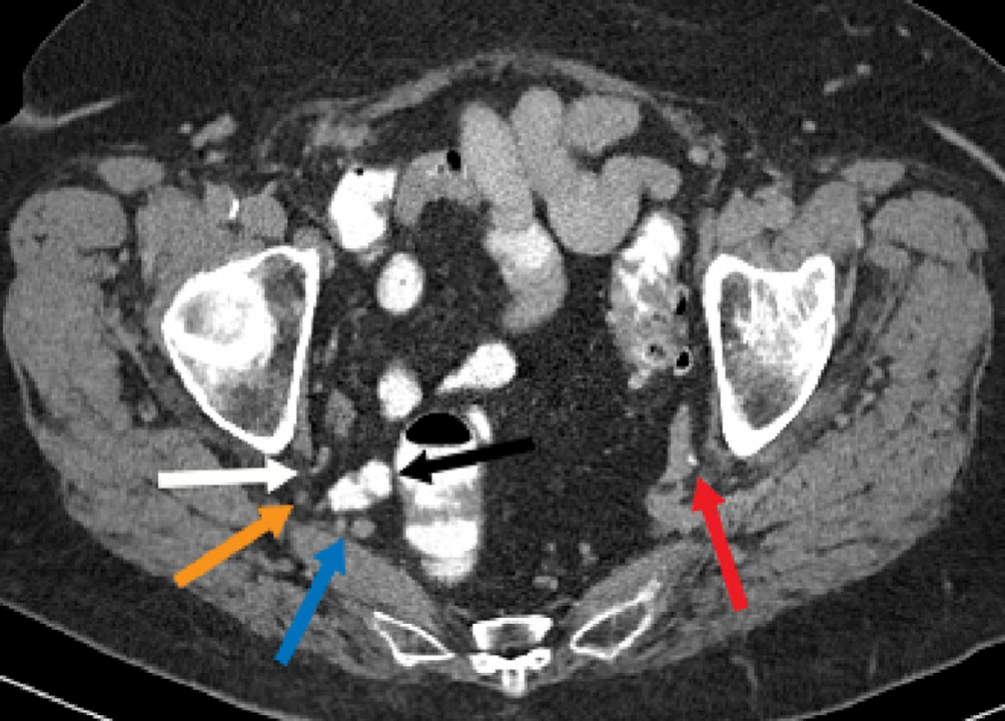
Axial CT image in the delayed phase shows the right ureter (white arrow) curving into the greater sciatic foramen in the infrapiriformis region. The sciatic nerve (orange arrow) and inferior gluteal vessels (blue arrow) are also seen at this infra-piriformis level. There is slight protrusion of the small bowel (black arrow) into the greater sciatic foramen. The left ureter (red arrow) has a somewhat posterior course near the sciatic notch, but subsequently coursed anteriorly towards the bladder (color version of figure is available online).
